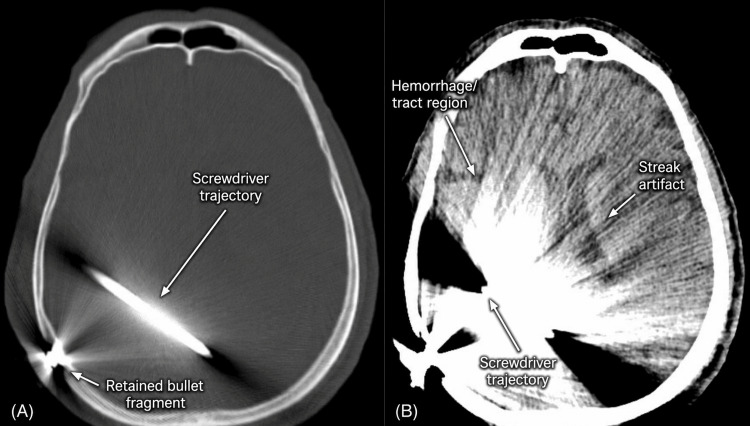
Admission CT demonstrating retained metallic foreign body and artifact-limited assessment (A) Admission axial noncontrast CT of the head on bone window demonstrating the screwdriver trajectory through the right frontoparietal calvarium and the retained bullet fragment from prior injury. (B) Admission axial noncontrast CT of the head on brain window demonstrating the screwdriver trajectory, marked streak artifact, and adjacent hemorrhage/tract region, limiting characterization of the surrounding brain parenchyma and extra-axial spaces
